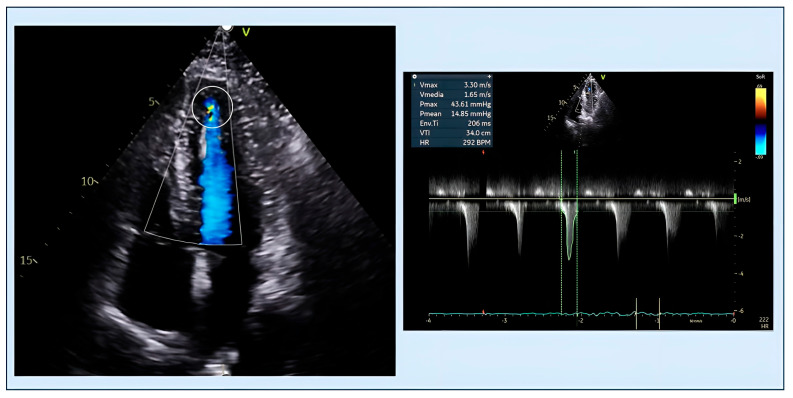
A 38-year-old male patient with ApHCM. Color Doppler imaging shows apical aliasing artifact (circled), suggesting localized obstruction. Continuous-wave Doppler aligned with the same jet confirms an apical systolic gradient, consistent with obstruction in ApHCM. ApHCM, Apical hypertrophic cardiomyopathy.
